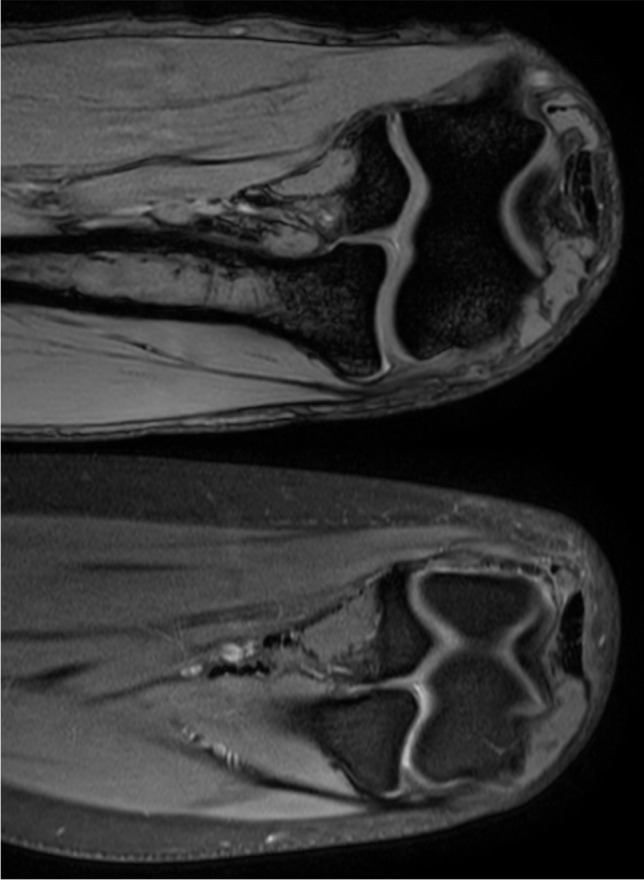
Views from the MRI scans of the synovial plicae of the elbow and their close anatomical areas
